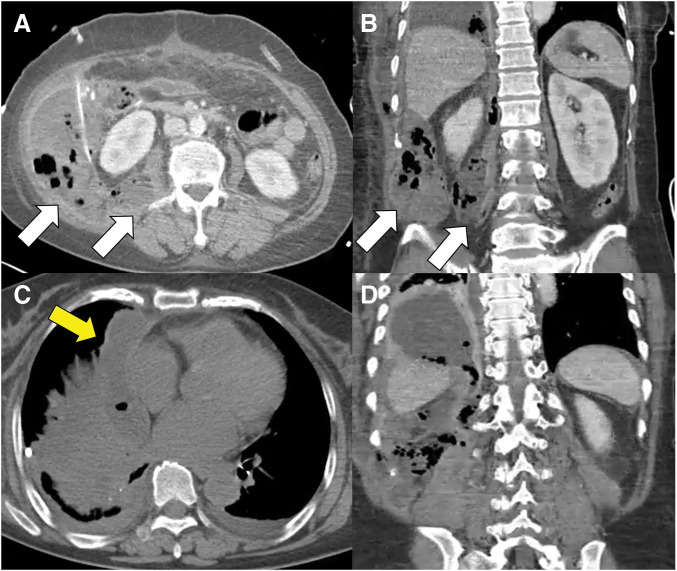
CT shows increased air-containing fluid around the hemicolectomy site (white arrows) (A and B). Worsening empyema persists despite drainage, with a newly visible paramediastinal gas-containing collection (yellow arrow) (C and D), suggesting mediastinitis.
